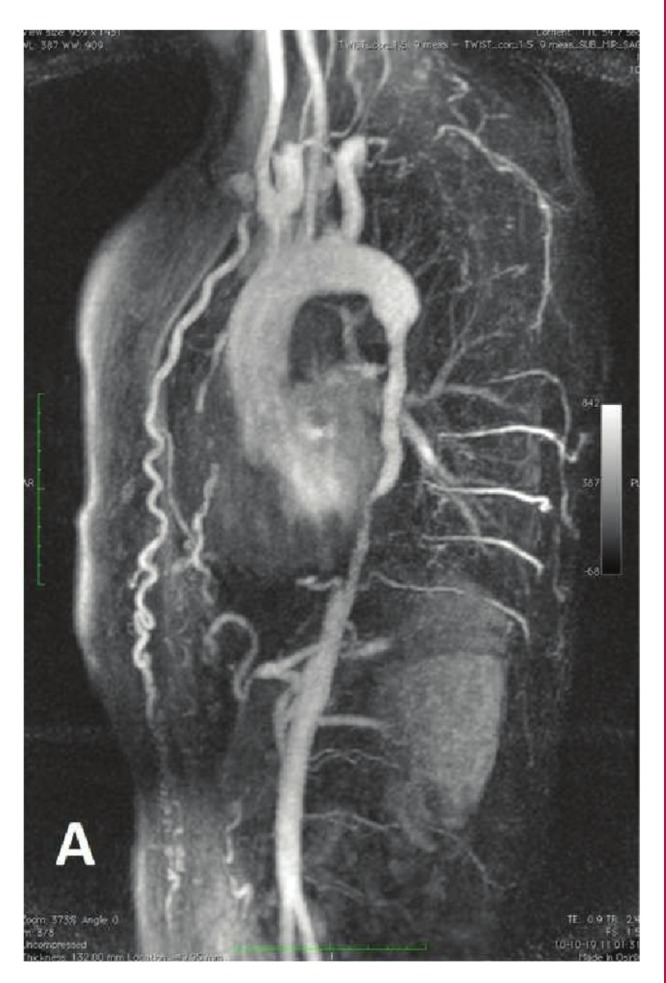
Pre-operative MRI. The 180-mm-long narrowing of the descending aorta with a critical (3-4 mm) coarctation. The excessively developed collateral circulation, mainly through intercostal branches and dilated left and right mammary arteries.
